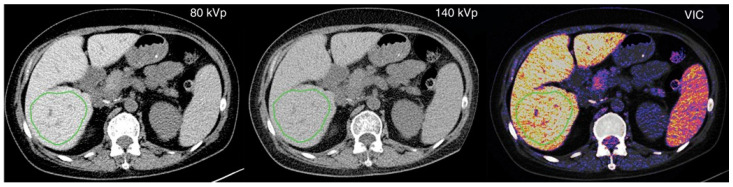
CT measurement in 54-year-old woman with myelodysplastic syndrome. Mean attenuation in liver was measured by placing largest possible freehand regions of interest at segments V and VI of right hepatic lobe. CT measurements were 117.8, 65.3, and 54.1 HU at 80 kVp imaging, 140 kVp imaging, and VICvirtual iron content imaging, respectively. Reproduced with permission from Luo XF et al. [18].
